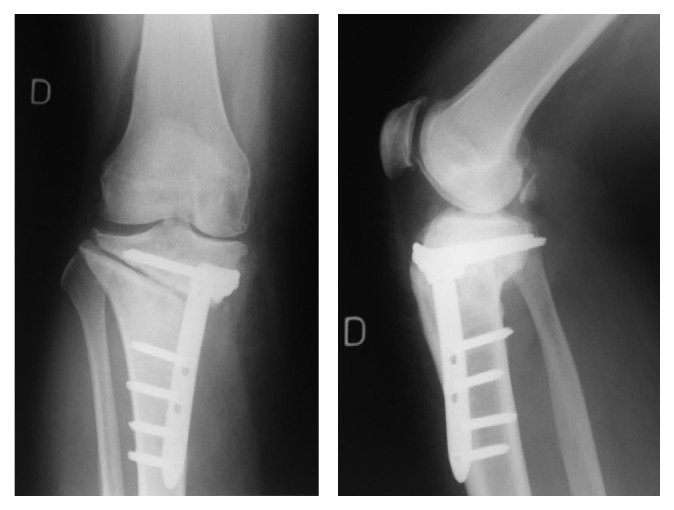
June 2011, 1-year after revision, postoperative X-rays showing fracture of the plate and translation of the proximal fragment of the HTO.
